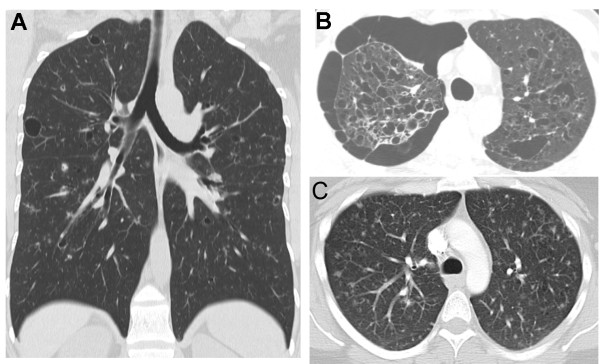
Chest CT findings in PLCH. A) Coronal chest CT image from a 36-year-old smoker with biopsy-proven PLCH demonstrating a combination of large and small lung cysts and pulmonary nodules, distributed predominantly in upper and mid-lung zones. B) Chest CT section of a 35-year-old smoker with biopsy-proven PLCH showing extensive bilateral upper lobe cystic lung changes and a medium-sized pneumothorax in the right thoracic cavity. C) Chest CT of a 40-year-old smoker with biopsy-proven PLCH showing a combination of diffuse nodular and cystic abnormalities in both lungs.
